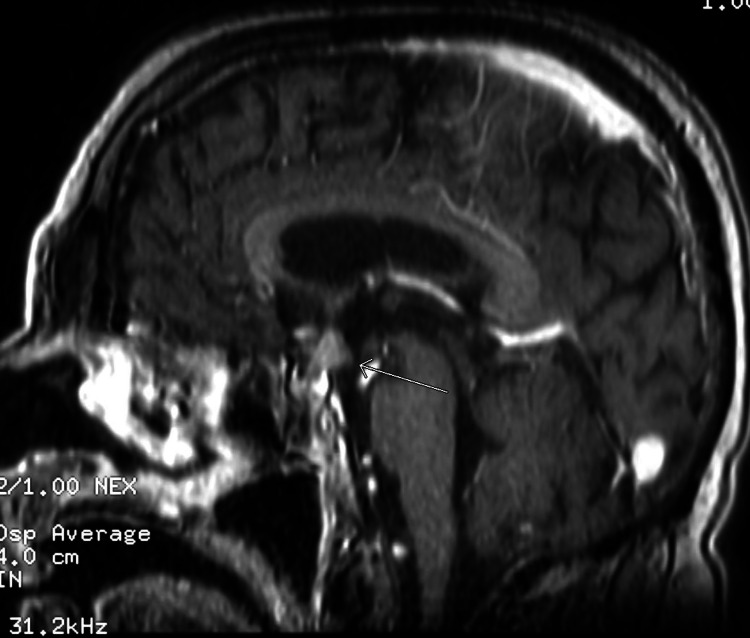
MRI of the brain (sagittal view) showing a contrast-enhancing lesion of the optic chiasm
